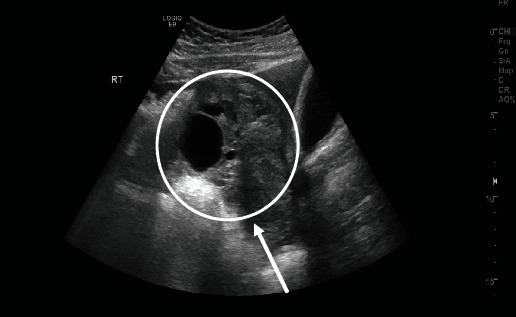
Pelvic sonography of the right ovary, visualizing an ectopic pregnancy.
